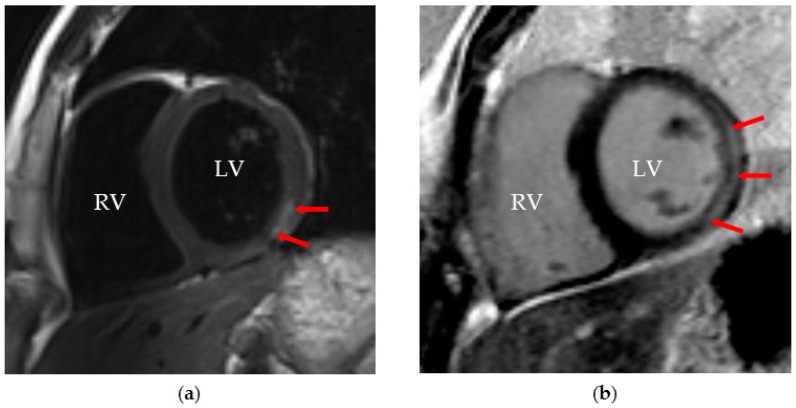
A 15-year-old boy with Epstein–Barr virus myocarditis: (a) a short-axis T2-weighted image demonstrating focal myocardial oedema (red arrows); and (b) a short-axis T1-weighted late gadolinium enhancement image (red arrows). RV, right ventricle; LV, left ventricle.
